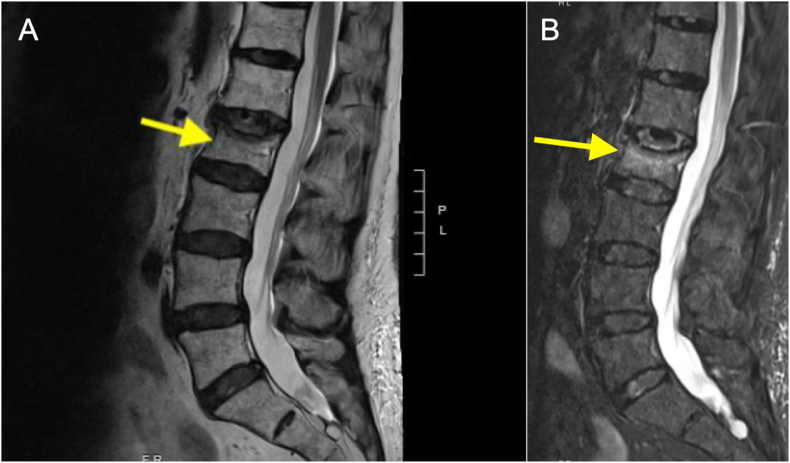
L2 vertebral compression fracture visualized using MRI sequences. (A) T2-weighted MRI with an arrow pointing to the fracture site. (B) STIR sequence with an arrow highlighting the bright signals indicative of edema/inflammation at the corresponding fracture location.
